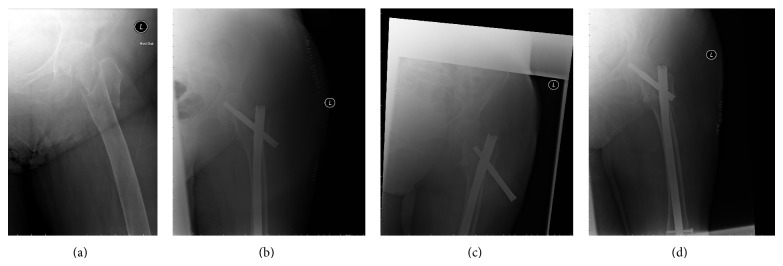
(a) 83-year-old female with proximal femoral fracture (AO/OTA type 31-A3). (b) Immediate postoperative radiograph. (c) Radiograph 15 months after surgery showing the backout. (d) Radiograph after revision of the lag screw.
